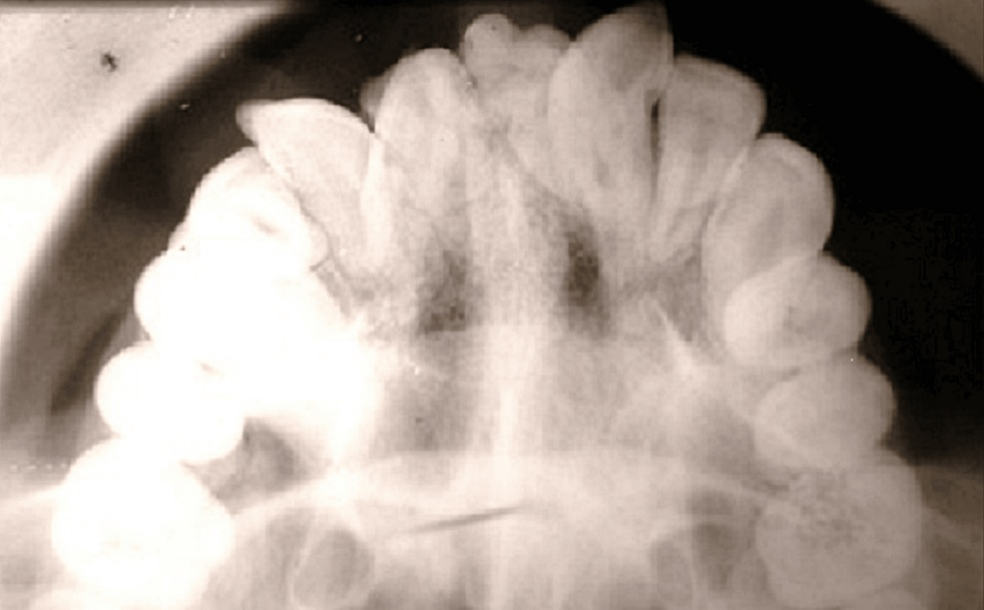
Occlusal radiograph showing tooth anomalies.
